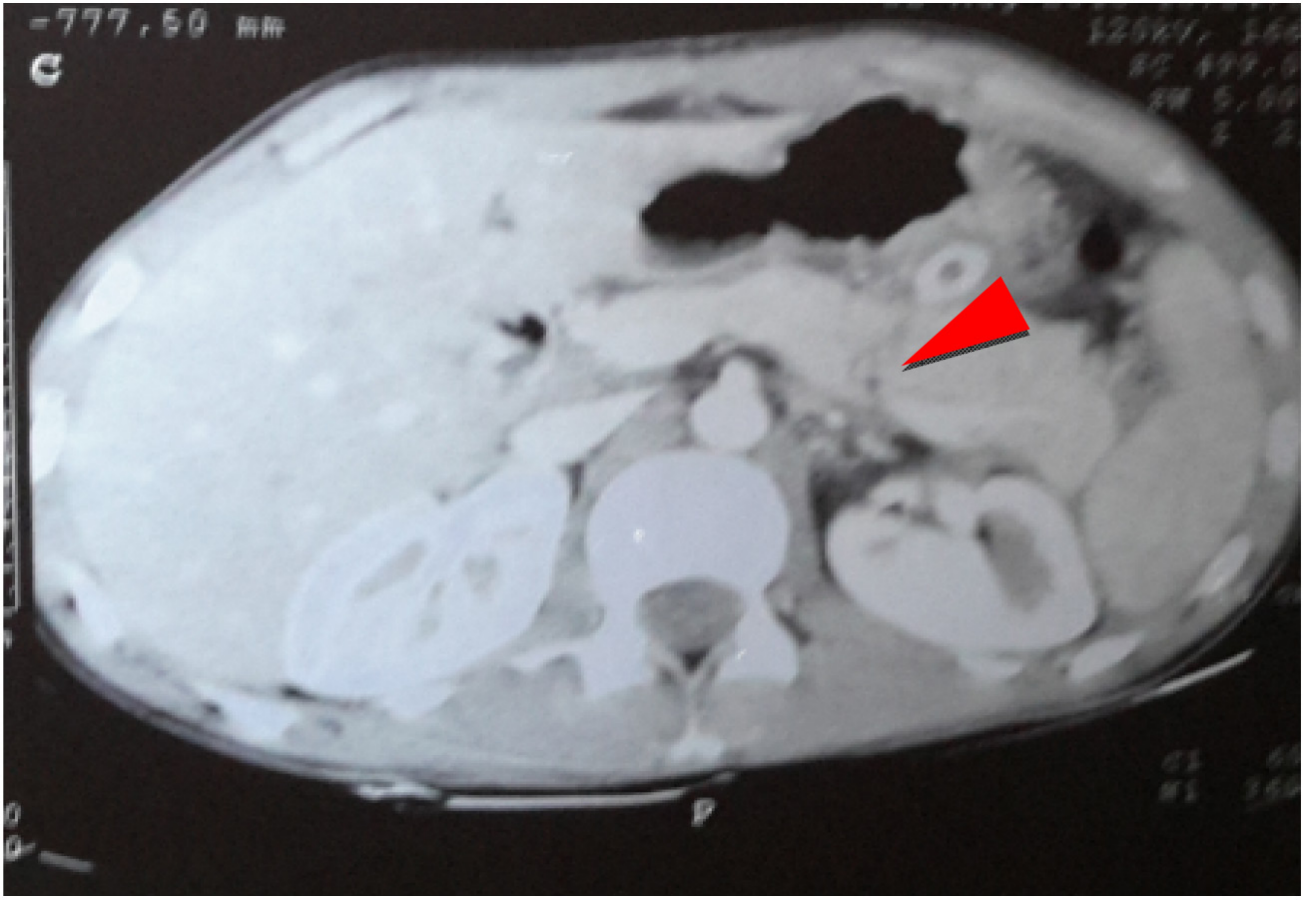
CT scan showing pancreatic body transection involving the duct (Red arrow), with distal pancreatic edema
